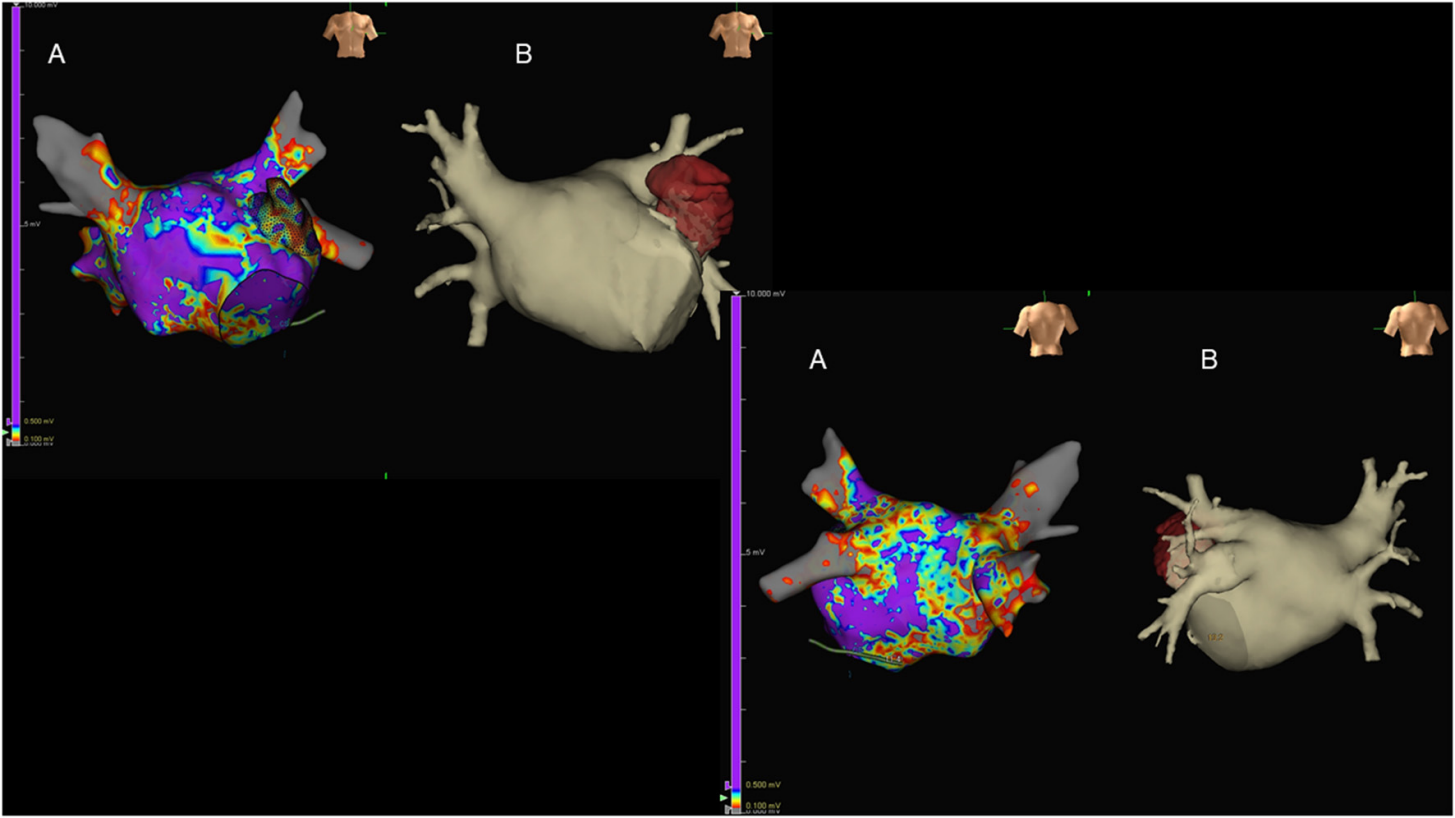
Antero-posterior (upper-left images) and postero-anterior (lower-right images) views of the left atrium (LA) in a patient with long-lasting atrial fibrillation submitted to endocavitary ablation. Three-dimensional (3D) LA anatomy was built by means of EAM [(A); Ensite Precision, St. Jude Medical, USA], aided by volume-rendered CT coronary angiography (CTCA) images (B). Of note, LA voltage maps were superimposed on the reconstructed 3D LA volume, showing widespread LA disease with diffusely reduced voltages evident.
